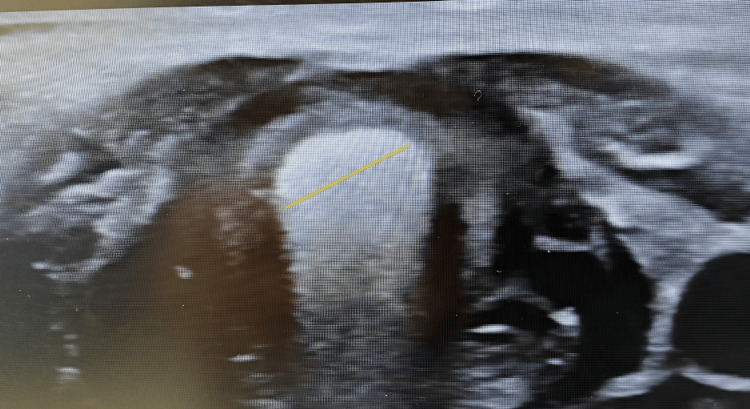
Subglottic airway measurement method with ultrasonography Yellow line: Minimal transverse diameter of subglottic airway measurement
